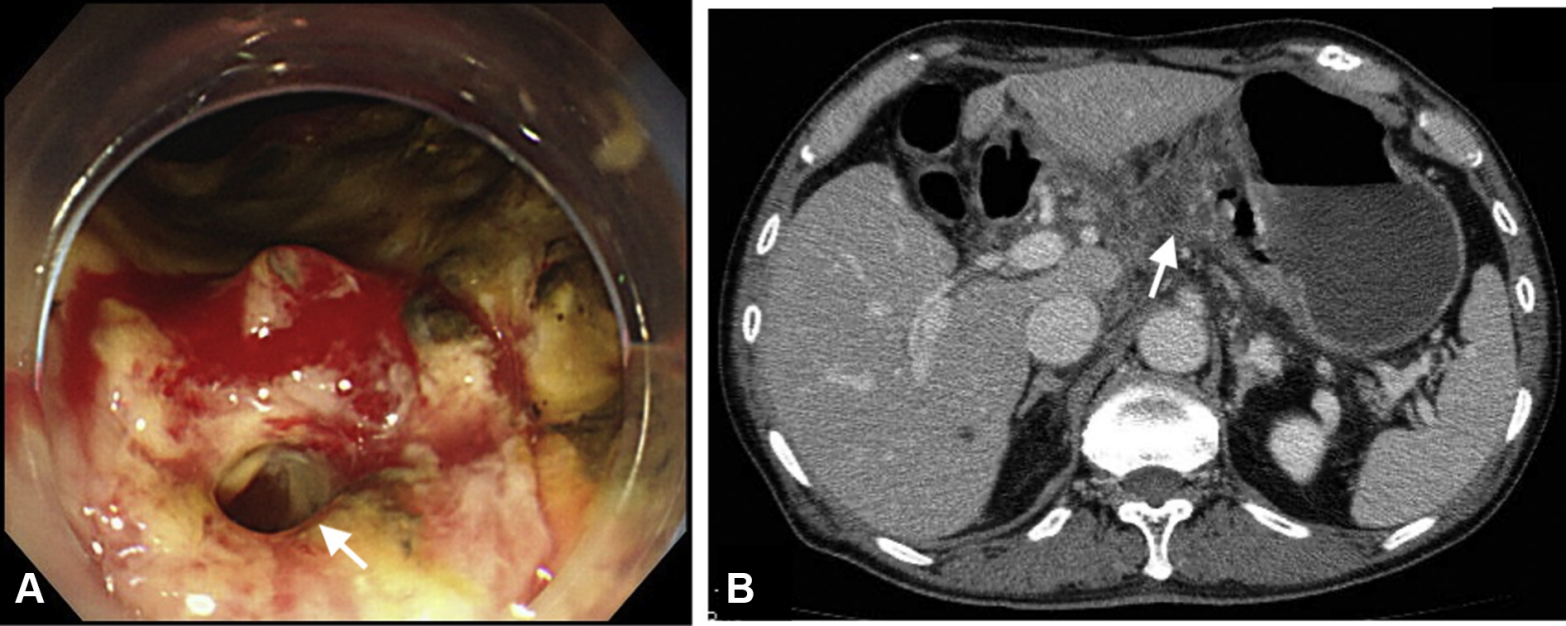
Delayed perforation occurred on postoperative day 5. A, A fistula (*white arrow*) in the post–endoscopic submucosal dissection ulcer was observed. B, Abdominal CT revealed fluid collection (*white arrow*) around the lesser curvature of the stomach.
